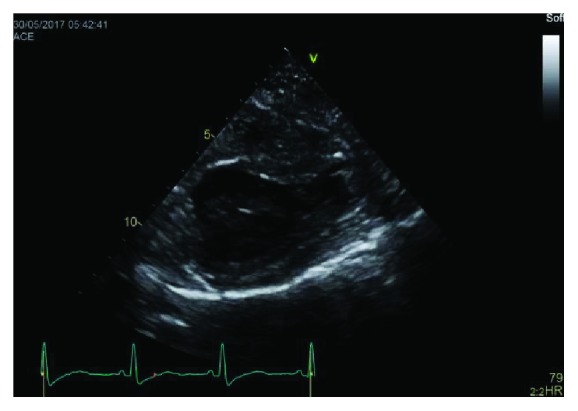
Parasternal short axis showing basal and apical akinesis with preserved contractility of the mid ventricular segments.
